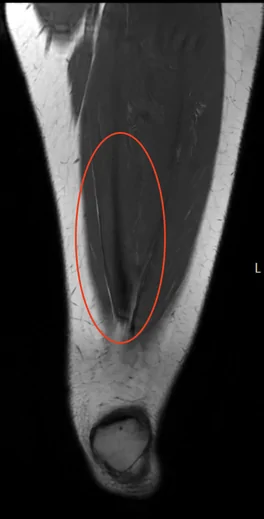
Left knee. Coronal T1‐weighted MRI scan of the distal thigh showing the anatomical course of the rectus femoris tendon. Within the red circle, the rectus femoris tendon can be identified between the 2 muscle bellies, confirming its superficial and central position within the quadriceps tendon complex. (MRI, magnetic resonance imaging.)
